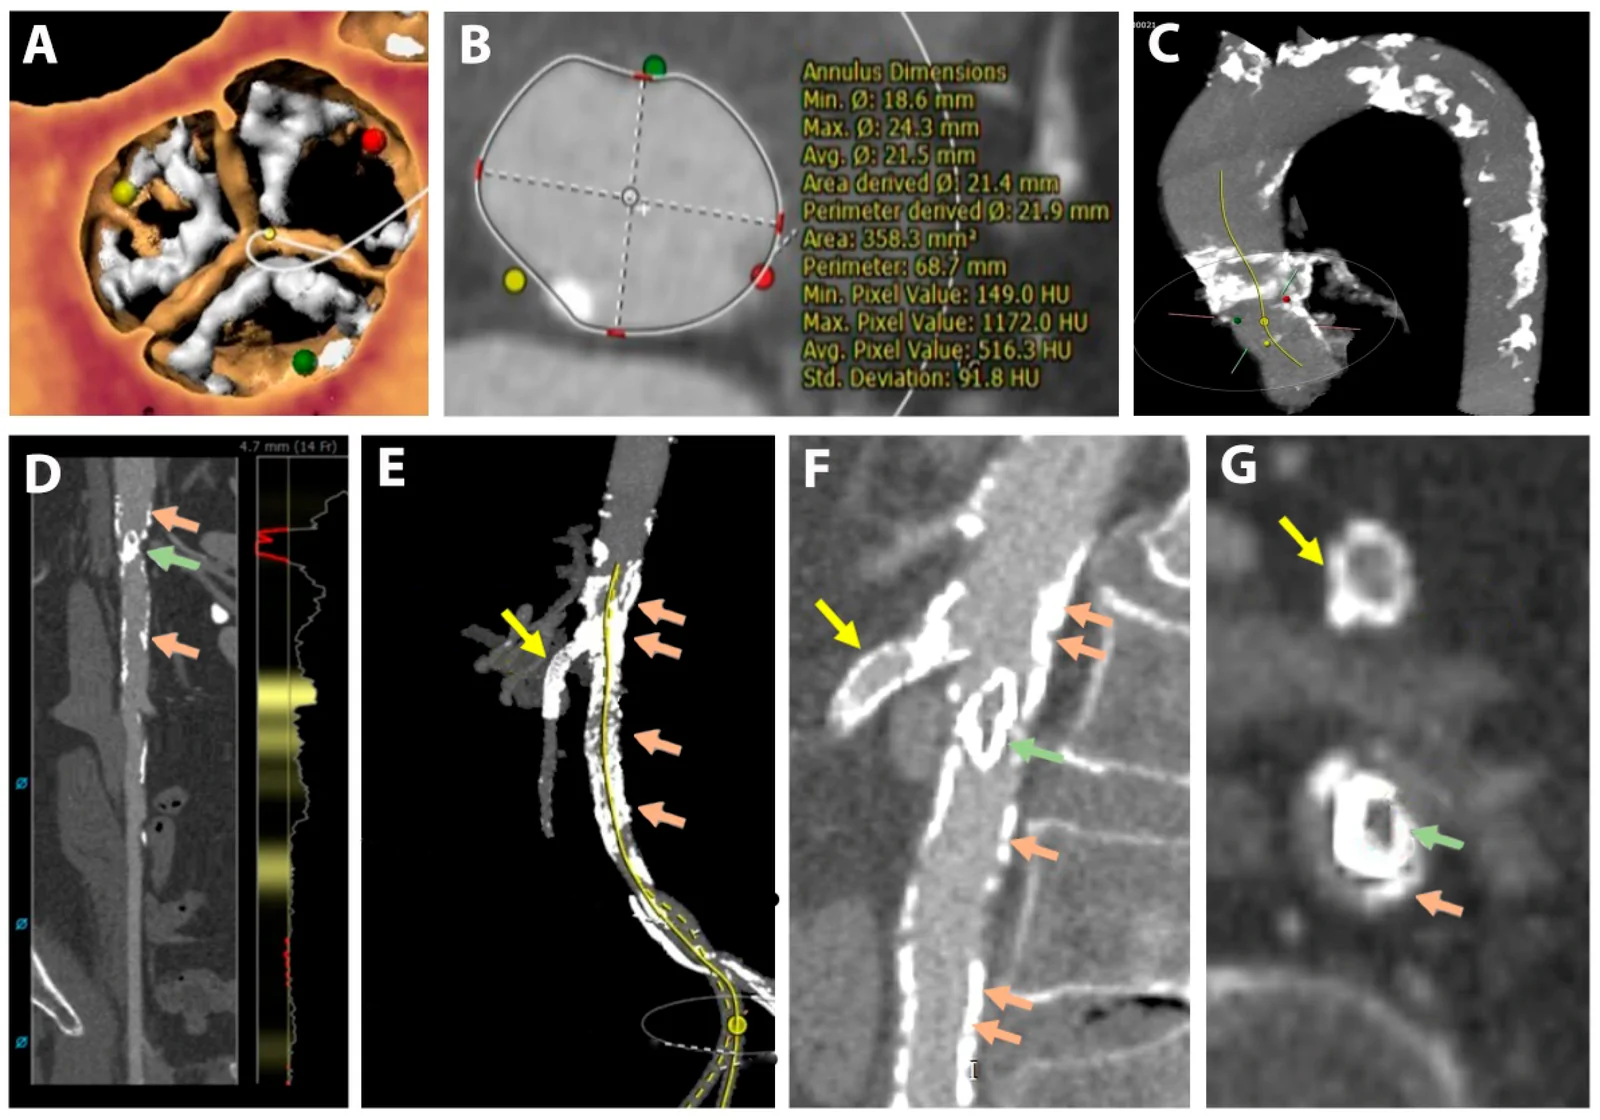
Preprocedural planning computed tomography. (A) Three-dimensional reconstruction of the heavily calcified tricuspid aortic valve. (B) Aortic annular measurements compatible with implantation of a 23-mm balloon-expandable valve. (C) Multiplanar reconstruction of LVOT, STJ, aortic arch and descending thoracic aorta calcification. (D) Assessment of the vascular access route demonstrating extensive calcific atherosclerosis of the abdominal aorta with focal infrarenal aortic stenosis and migrated stent fragment; the iliofemoral vessels were considered suitable for transfemoral access. (E) Multiplanar reconstruction demonstrating the calcified abdominal aorta and in situ SMA stent. (F) Close-up sagittal view demonstrating the in situ SMA stent and migrated stent fragment within the abdominal aorta, and extensive abdominal aortic calcification. (G) Axial CT images demonstrating the in situ SMA stent and the migrated stent fragment within the abdominal aortic lumen. Stent fragment—green arrow; SMA and residual stent—yellow arrow; aortic calcifications—orange arrow. Abbreviations: SMA, superior mesenteric artery; STJ, sinotubular junction; LVOT, left ventricular outflow tract.
